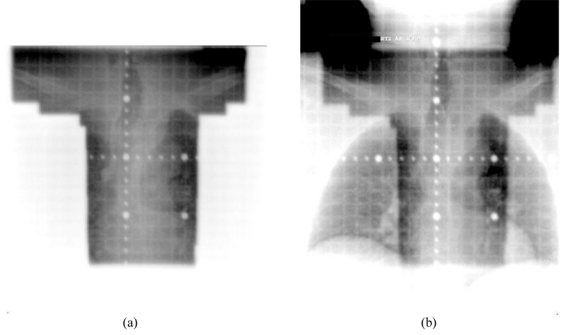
kV X-ray source and flat panel detector fixed at 90° to treatment beam of a linear accelerator for image guidance with kV cone beam CT.
